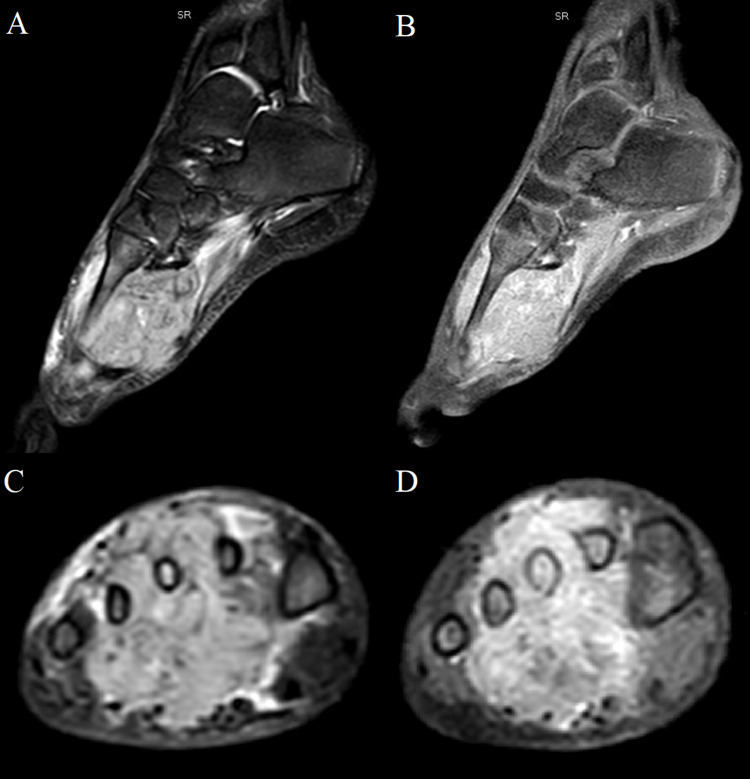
MRI of the right foot with and without contrast. (A) Sagittal STIR sequence. (B) Sagittal postcontrast T1 fat-suppressed sequence. (C) Axial STIR sequence. (D) Axial postcontrast T1 fat-suppressed sequenced. Note the large STIR hyperintense and avidly enhancing soft tissue mass centered in the metatarsal/intermetatarsal region with corresponding marrow signal abnormality.
